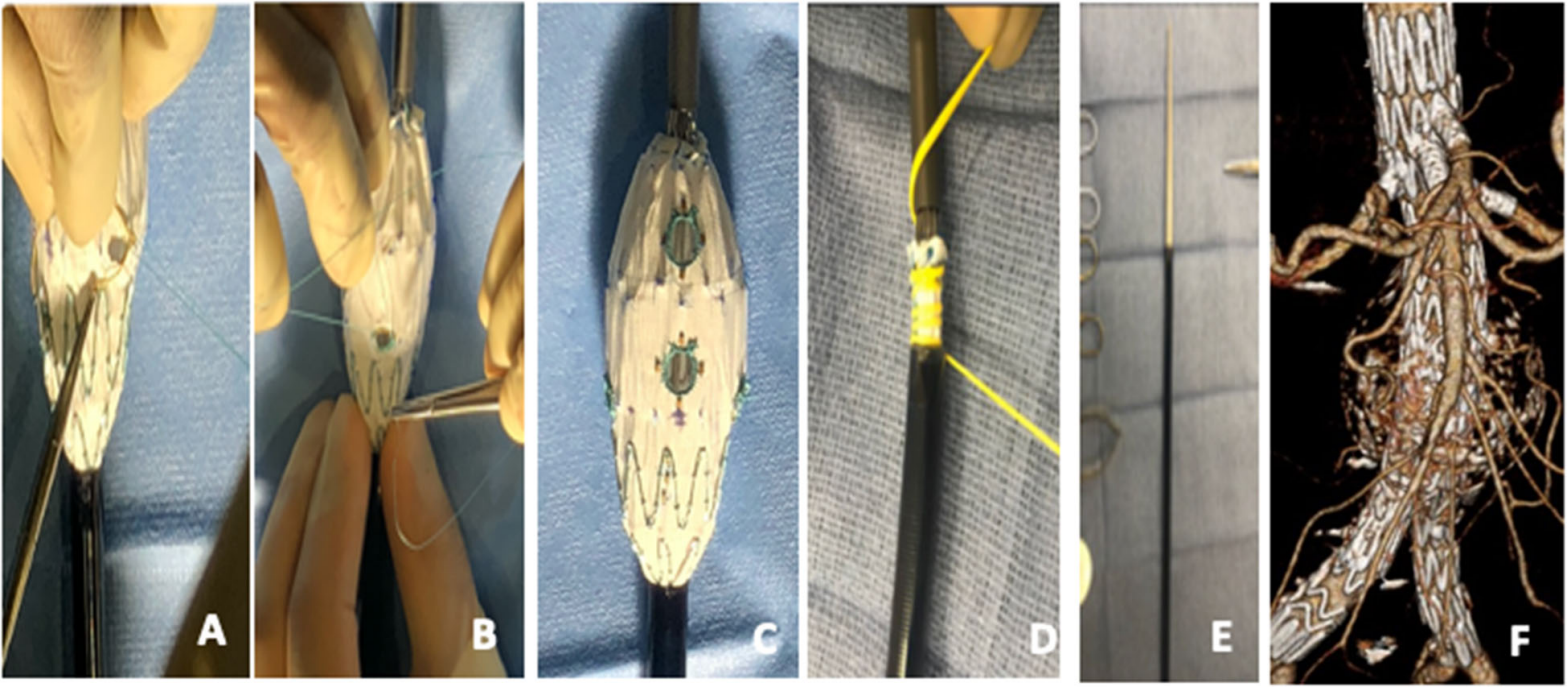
A-F. Steps involved in modification of the Zenith fenestrated stent graft (Zfen). The device is already constrained posteriorly and comes with 2 fenestrations created by the manufacturer to accommodate the celiac and superior mesenteric artery. Modification requires only partial deployment of the graft (A). Fenestrations are created and reinforced as described in Fig. 5C. (A, B, and C). The device is collapsed with silastic loops (D) and resheathed (E). Follow up post-operative 3D CTA confirm continued aneurysm exclusion and patency of target vessels. Rationale for device selection: Zfen is by far the easiest device to modify as it is already constrained and modification only requires addition of desired fenestrations and resheathing. This is our device of choice for all elective cases
